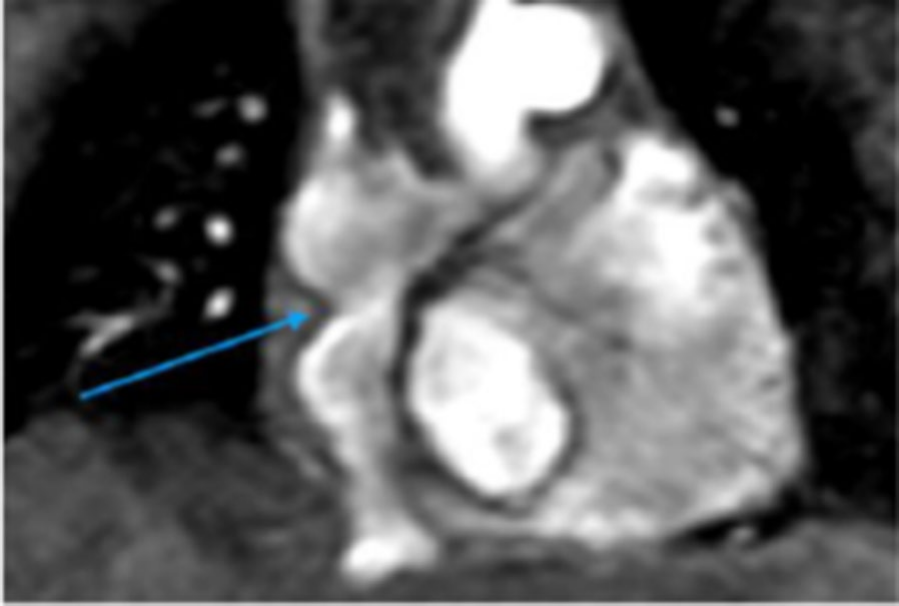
Still-shot MRI SSFP cine in the coronal view. Stenosis within the intra-atrial tunnel in a patient with a Fontan circuit (blue arrow). Re-used with permission from Fontan circulation in an adult: A guide for the radiologist. Arzanauskaite M, Nyktari E, Voges I. ESTI-ESCR 2018 / P-0103
